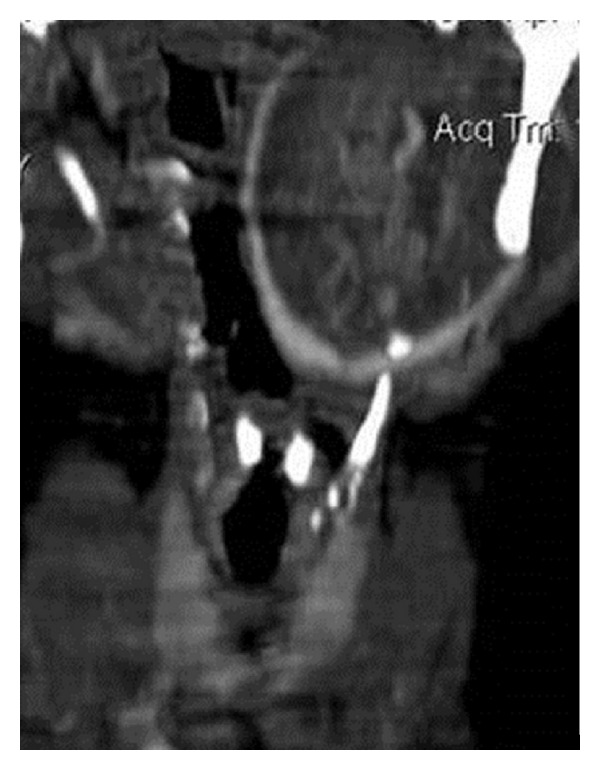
Coronal reconstructed CT image demonstrates splaying of carotid bifurcation by the mass.
